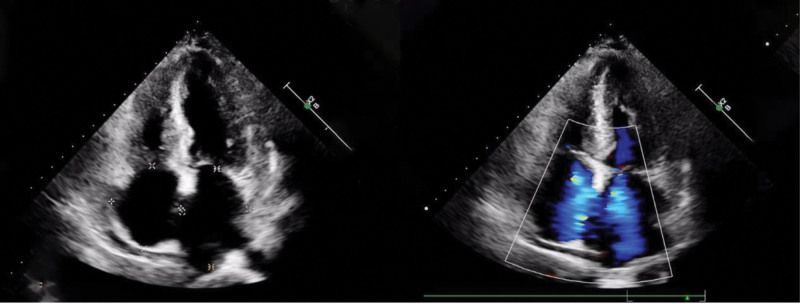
Echocardiography showed bilateral atrial enlargement.
